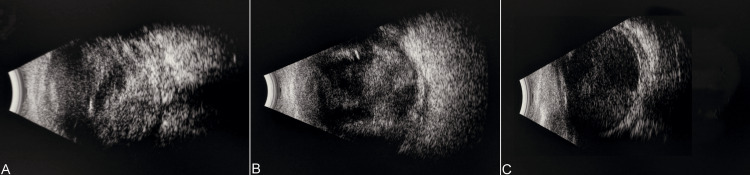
Serial B-scan ultrasonography of the left eye demonstrating progressive intraocular disorganization from acute vitritis to established phthisis bulbi (A) Day 3 after presentation: markedly hyperechogenic vitreous with early dense membranous echo organization, consistent with active vitritis; the retina remains attached in all four quadrants. (B) Day 7 after presentation: progressive organization of vitreous echoes with thickening of membranous formations and persistent diffuse vitritis; retinal attachment is maintained. (C) Six weeks after presentation: marked reduction in globe size with diffuse choroidal thickening, consistent with evolving phthisis bulbi.
